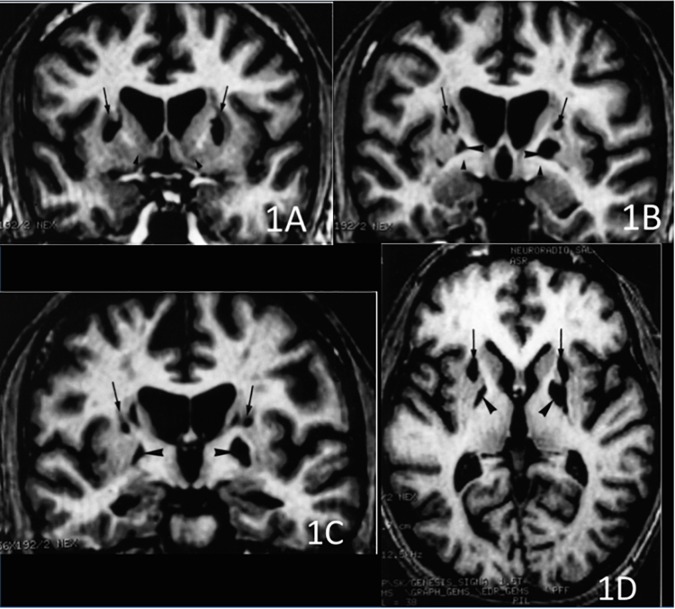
3D T1-weighted axial and coronal sections at 3 different rostro-caudal levels of the lesion of the basal ganglia. (A) Coronal section passing at the level of the head of the caudate nucleus and the ventral striatum. The lesions involved the dorsal striatum (arrows) while the ventral striatum was apparently spared (arrowheads). (B) Coronal section passing at the level of the anterior commissure. Infarction was located in the upper part of the putamen (arrows) and the globus pallidus (large arrowheads). The head of the caudate nucleus was atrophic. The ventral pallidum was spared (small arrowheads). (C) Coronal section passing at the level of the mammillary bodies. The lesion involved the upper part of both putamen (arrows) and the globus pallidus (arrowheads), bilaterally. (D) Axial section showing the rostro-caudal gradient of striatal lesion, which predominated in the rostral “associative” part of the striatum (arrows), and the lesion of the globus pallidus (arrowheads).
